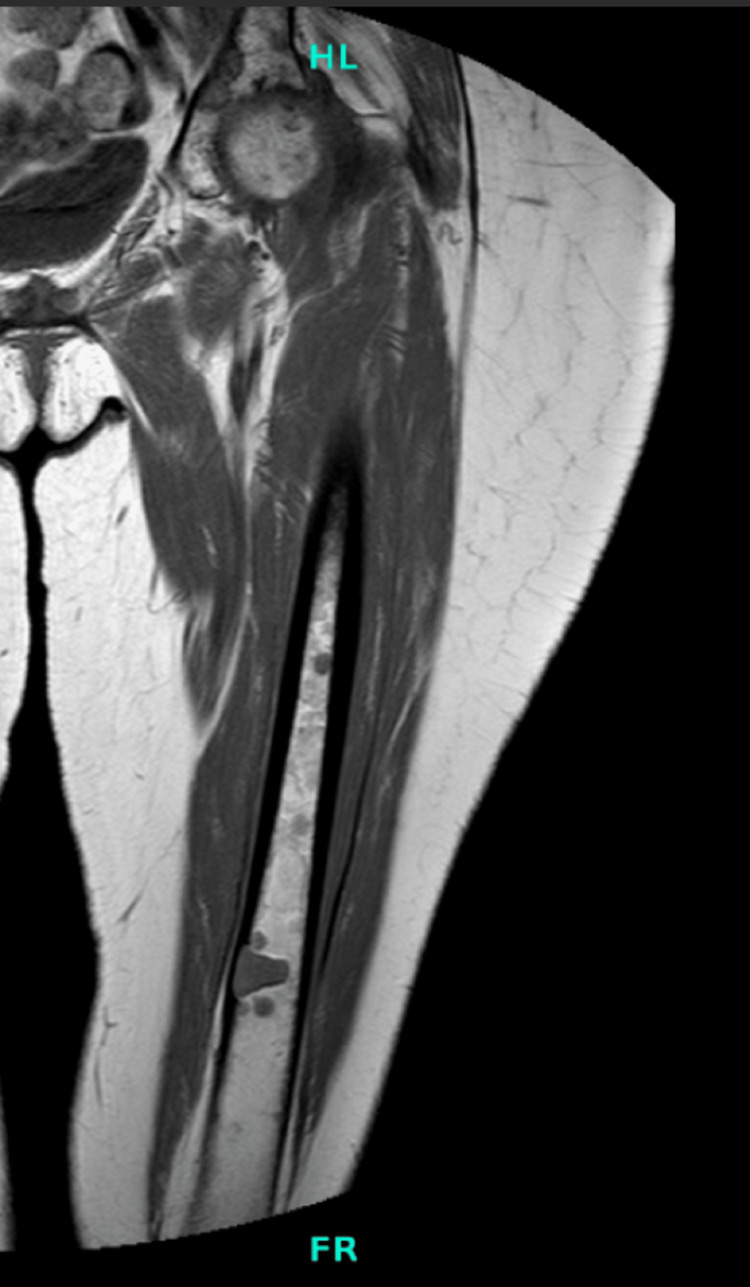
MRI of left hip demonstrating distal femur lesion MRI: magnetic resonance imaging
